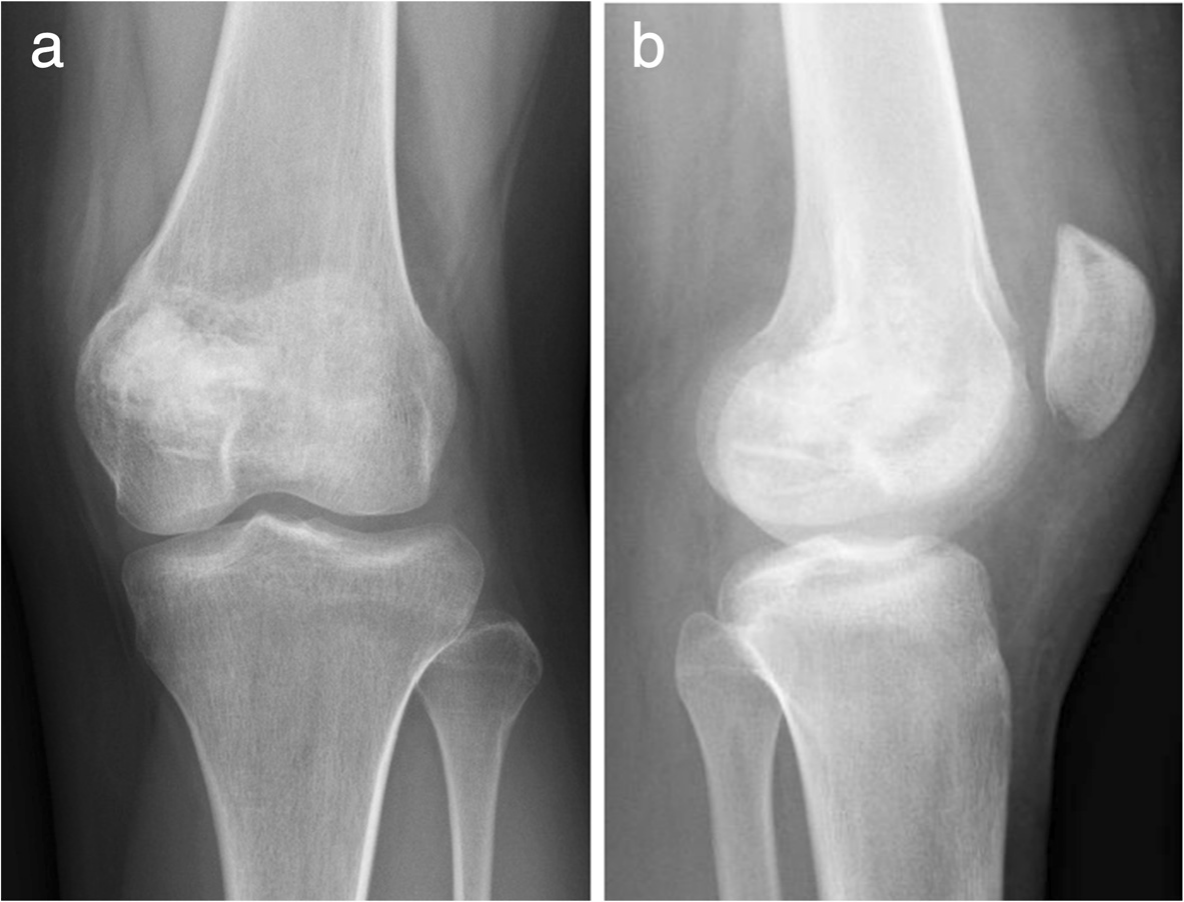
Radiographs at one-year follow-up, showing bone remodeling and consolidation (a,b).
